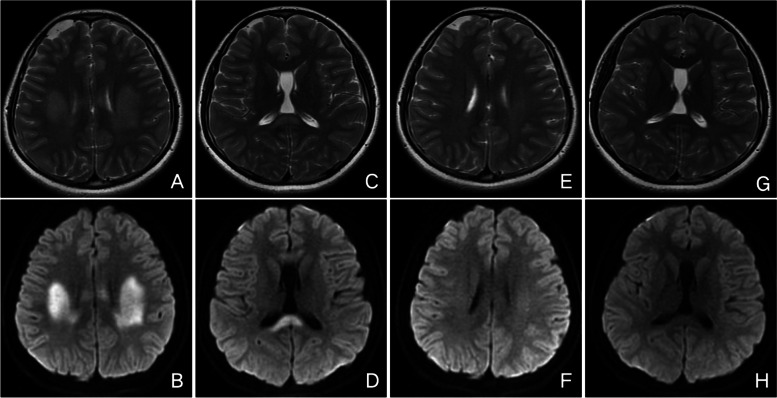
Cranial MRI of case 1 in 2018. Initial cranial MRI showed lesions in the entire corpus callosum and diffuse white matter on T2-weighted images (A,C) and DWI (B,D). Follow-up MRI on day 18 showed resolution of the lesions on T2 (E,G) and DWI (F,H)
